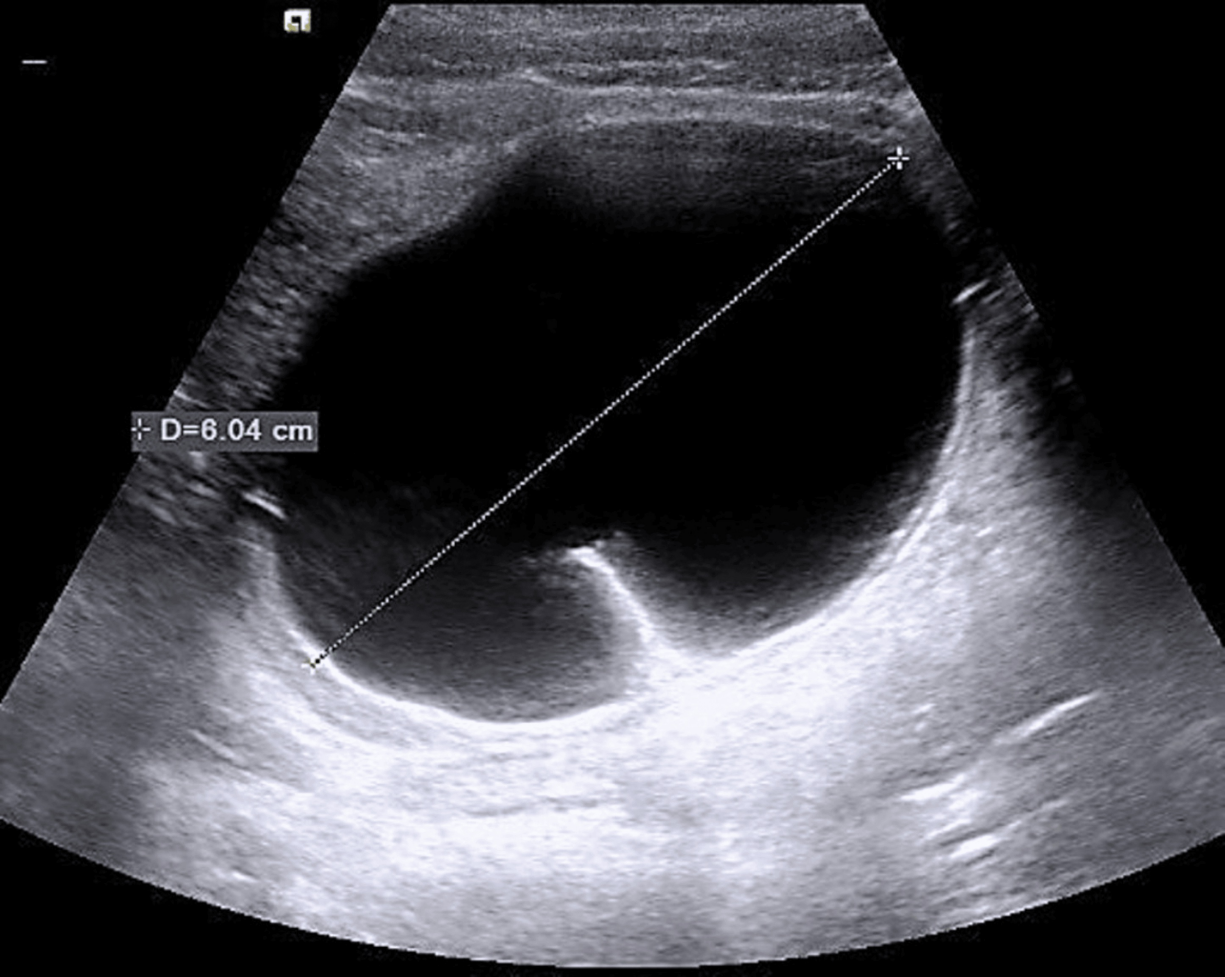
Abdominal ultrasound revealing the right-side ovarian cyst measuring 6 cm.
